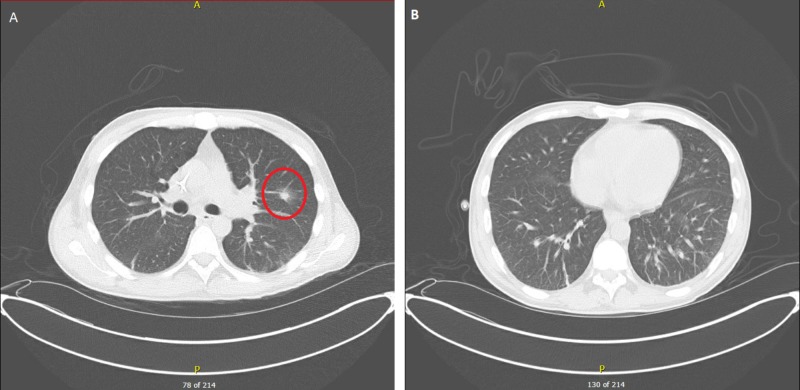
Lung computed tomography A. Red circle surrounding dominant subsolid nodule in the left upper lobe measuring approximately 10 x 8 mm with surrounding ground glass opacities; B. bibasilar reticular and ground glass opacities.
